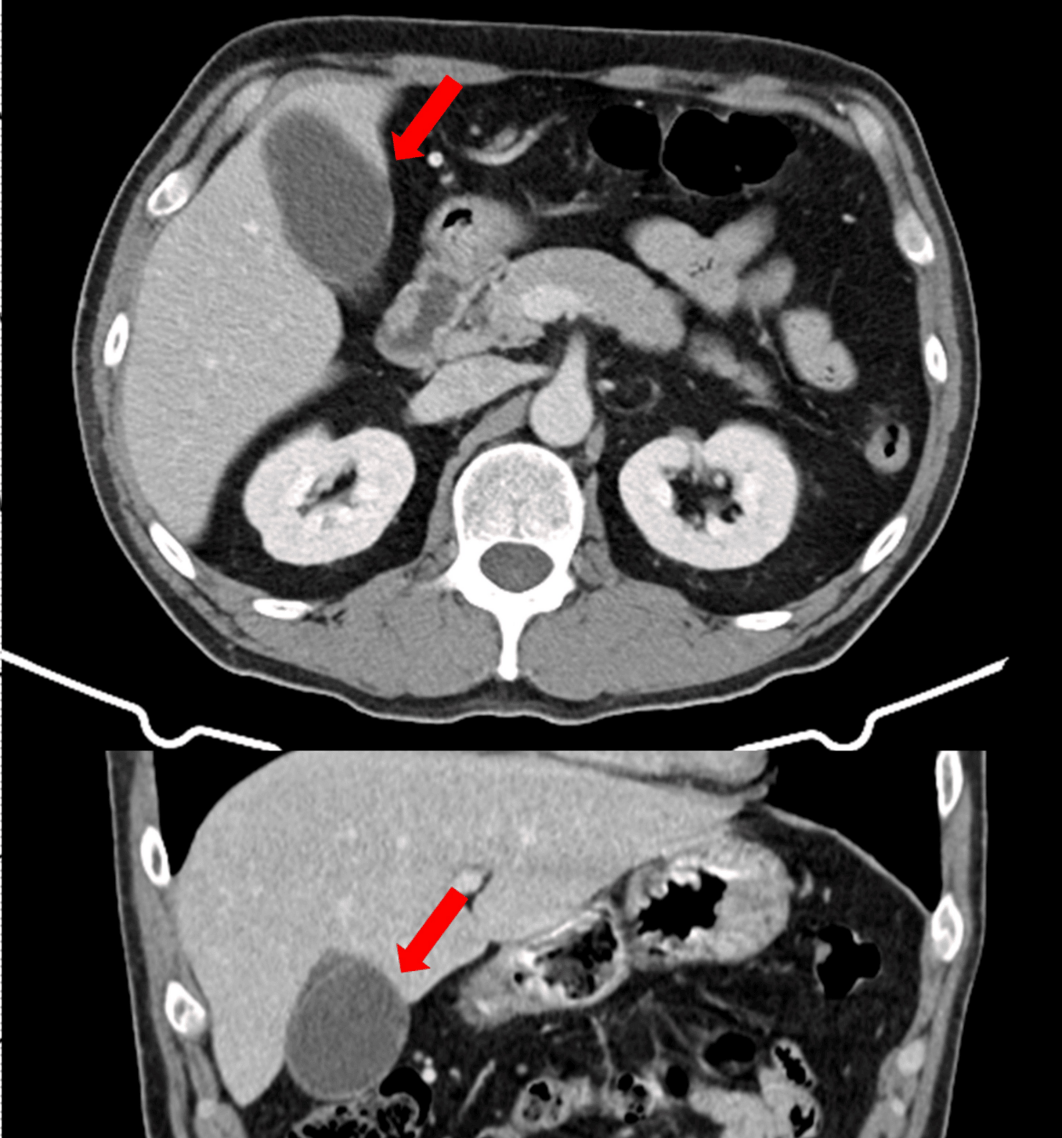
CT findings in Case 2 Abdominal CT showing gallbladder enlargement and wall thickening, consistent with acute calculous cholecystitis (red arrow).
